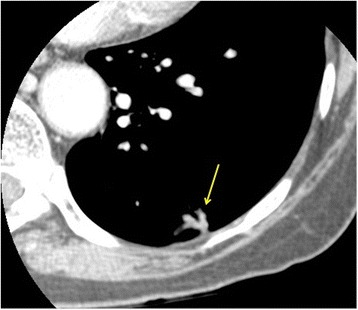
Axial CT shows a ‘polygonal’ nodule with no central spherical component that is also not suitable for DCE-CT
